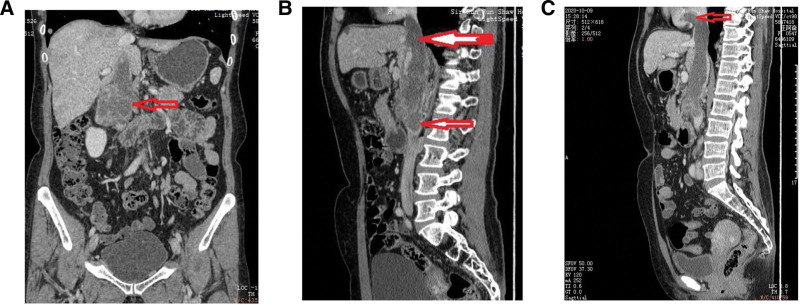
(A) Contrast-enhanced CT coronal view of the abdomen showed the primary lesion of the inferior vena cava. (B–C) Contrast-enhanced CT coronal view of the abdomen showed that the carcinoma thrombin of IVC extended to the right atrium. CT = computed tomography, IVC = inferior vena cava.
